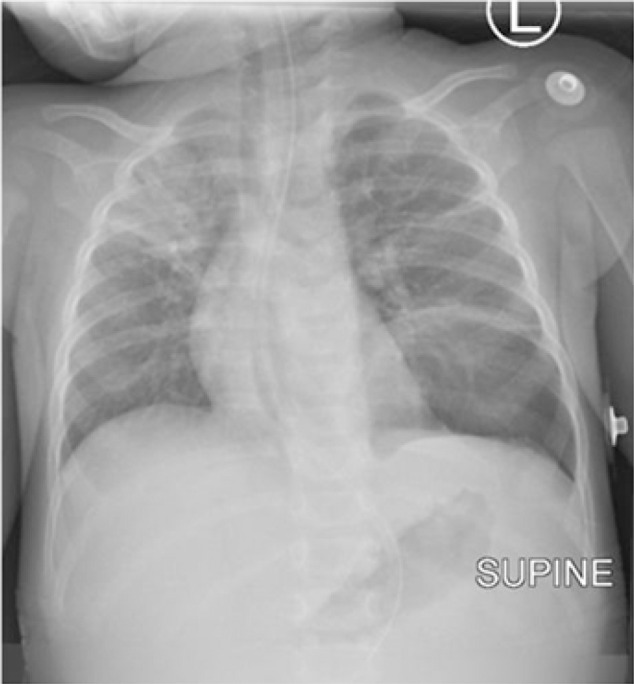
Post-operative Supine Antero-Posterior Chest X-Ray Chest x-ray, no longer shows protruding stomach in the left hemithorax. Consolidation of the anterior segment of the right upper lobe noted in keeping with pneumonia. Atelectatic bands seen in the left mid zone.
